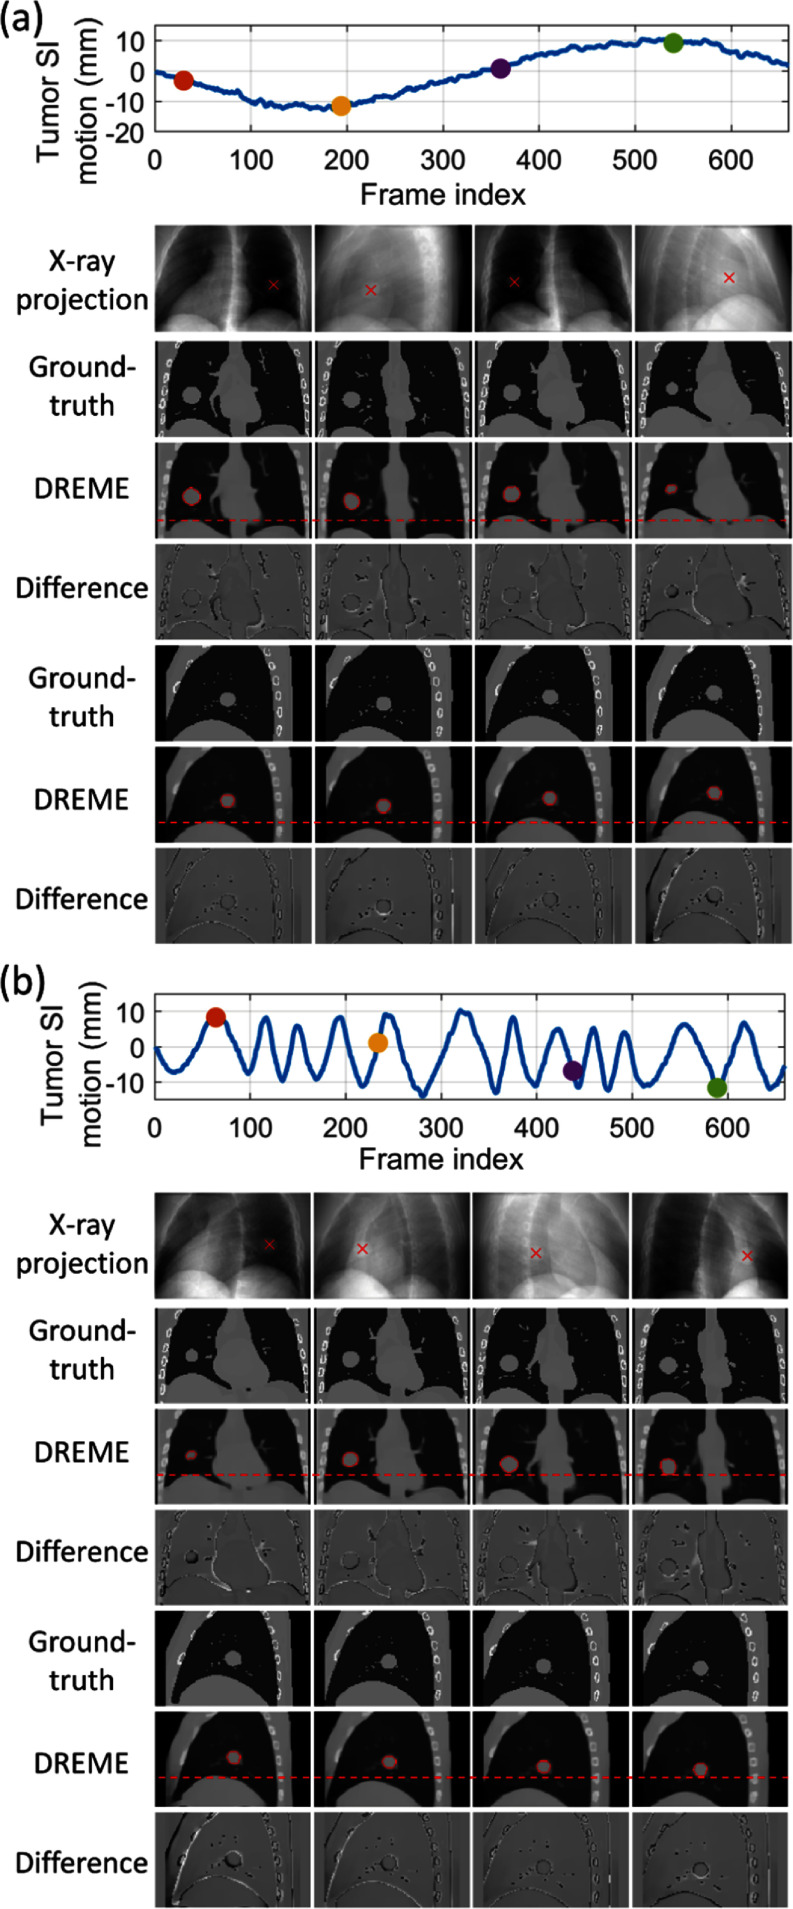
Examples of real-time CBCTs and lung tumor motion solved by DREME for the XCAT study: (a) X5 scenario and (b) X6 scenario. The training scenario was X3. The first row shows the tumor motion curves along the SI direction, with the dots indicating the time points selected for plotting. The second row presents the onboard x-ray projections at the selected time points, and the ‘×’ symbols indicate the solved tumor center-of-mass positions projected onto the projections. In the following rows, real-time CBCTs of the selected time points are compared against the ‘ground-truth’ CBCTs, with the difference images calculated. The estimated tumor contours (red) are also presented for each selected time points.
